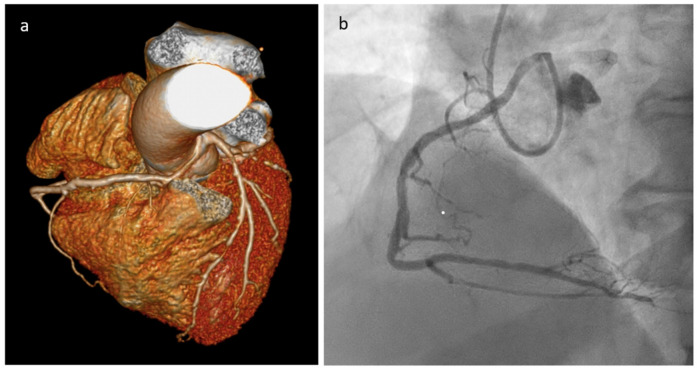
A 45-year-old male patient with a right coronary artery arising from the left coronary sinus: computed tomography volume-rendering image (a) and coronary angiography (b).
